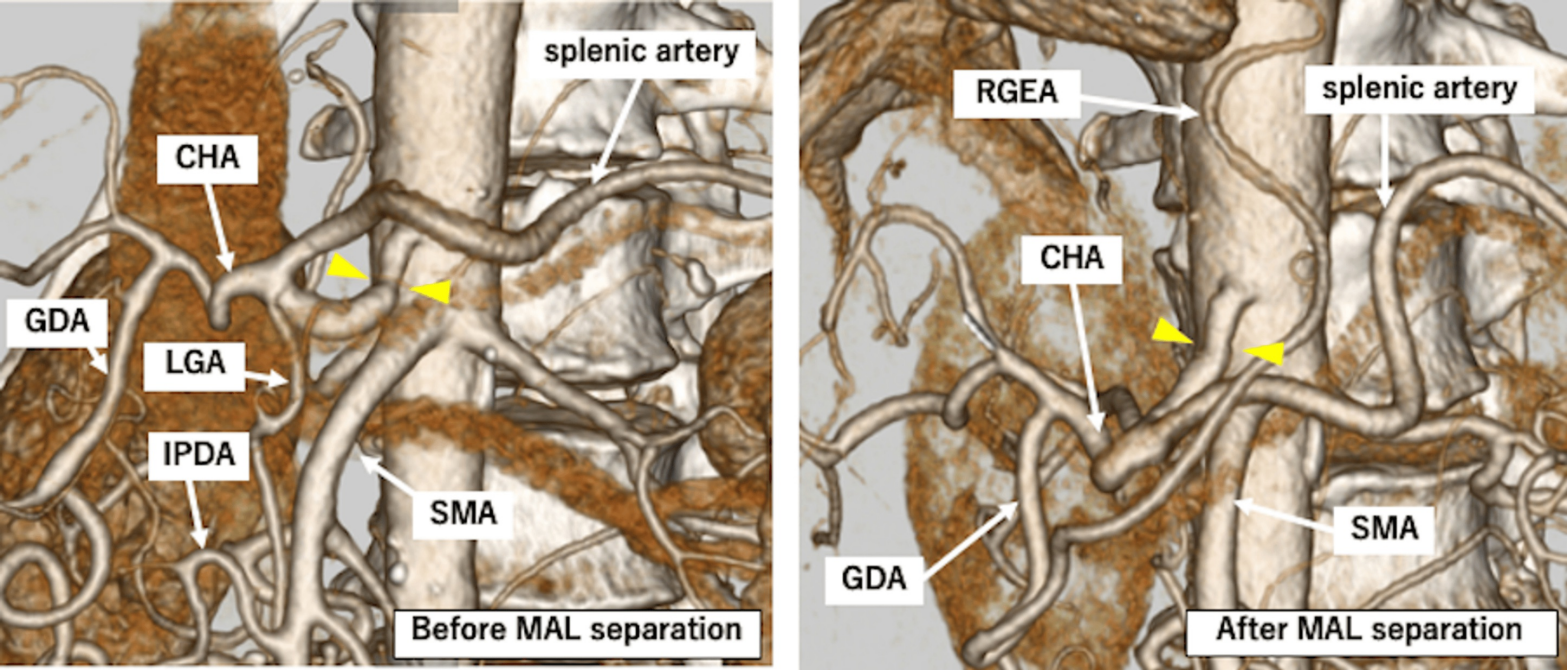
Contrast-enhanced CT of the thorax and abdomen. 3D-CT: (▲) CT images before and after MAL resection are shown. No calcification is present in the CA. (MAL: median arcuate ligament, CA: celiac artery, SMA: superior mesenteric artery, CHA: common hepatic artery, GDA: gastroduodenal artery, LGA: left gastric artery, IPDA: inferior pancreaticoduodenal artery, RGEA: right gastroepiploic artery)
